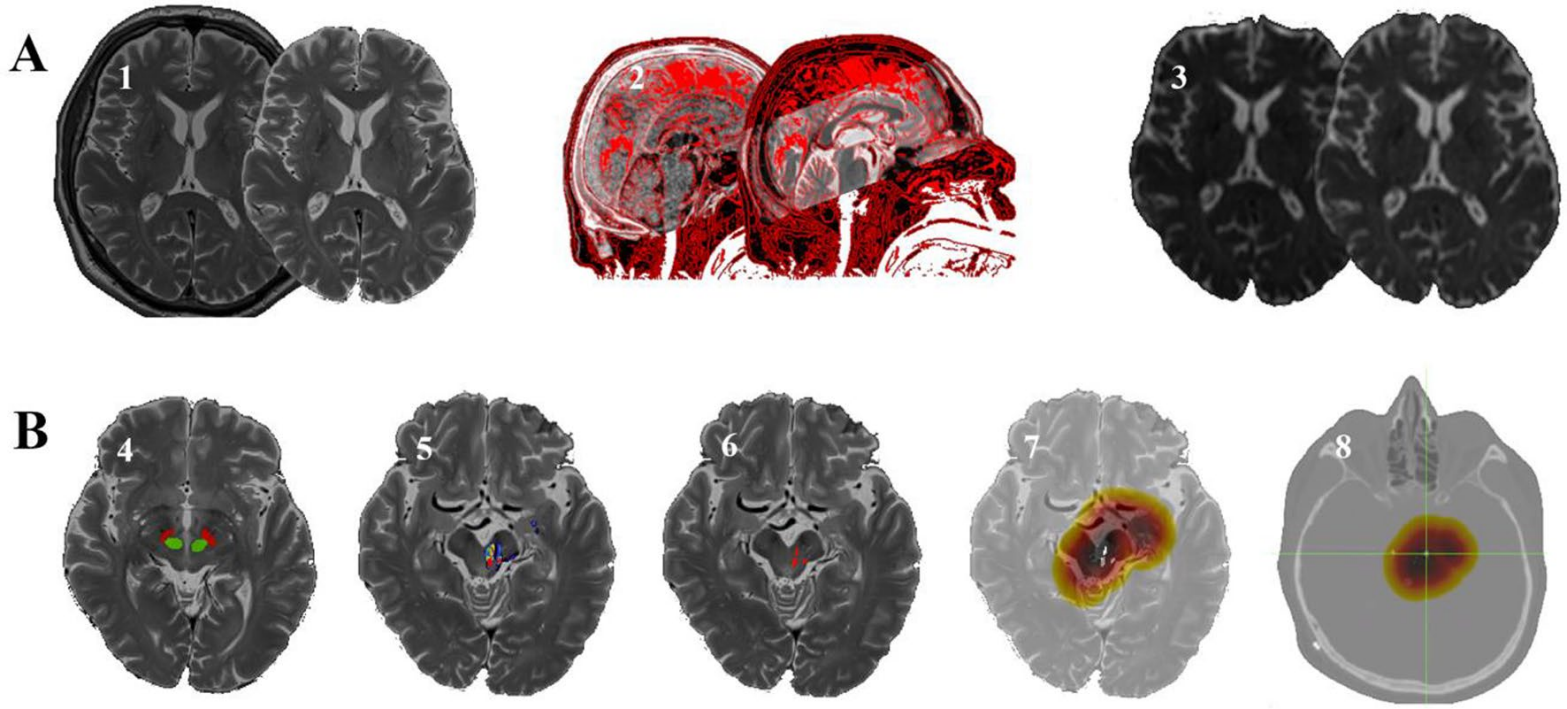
A Different steps of preprocessing: From left to right: (1) brain extraction, (2) co-registration of CT and MRI, (3) correction of eddy-current induced errors, proband movement errors, and susceptibility-induced errors. B Different steps of processing: (4) definition of seed and target regions, (5) calculation of probability maps, (6) tract binarization, (7) calculation of distance maps, and (8) export of distances based on the distance maps for the pole coordinates of the DBS electrode
